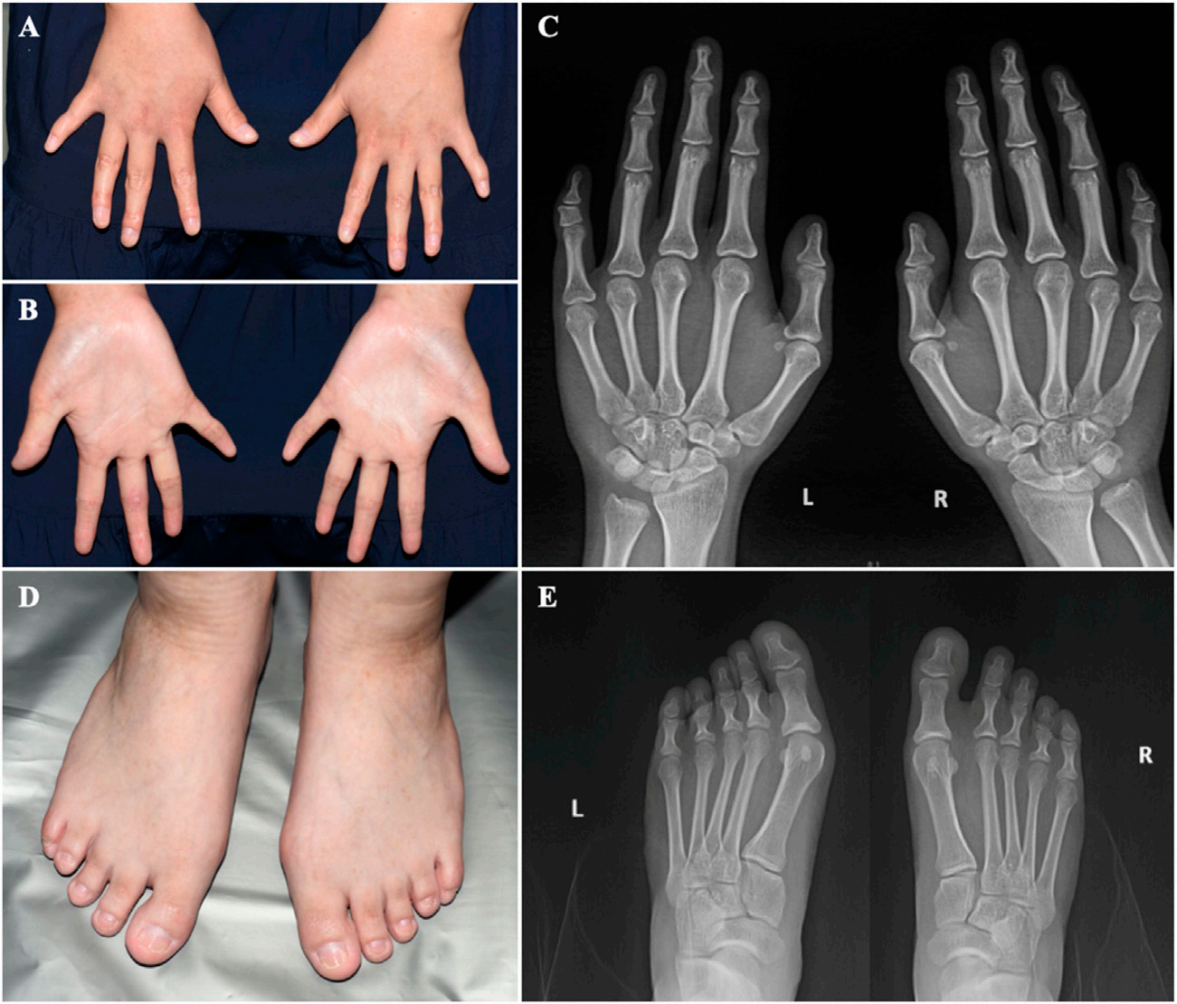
The hands and feet of the patient (A) Bilateral clinodactyly of the fifth finger. (B) Palmar hyperlinearity. (C) Radiograph of the hands showing clinodactyly of the fifth fingers. (D) Dystrophic nail plates and horizontal grooves along the length of the nails. (E) Radiograph of the feet.
